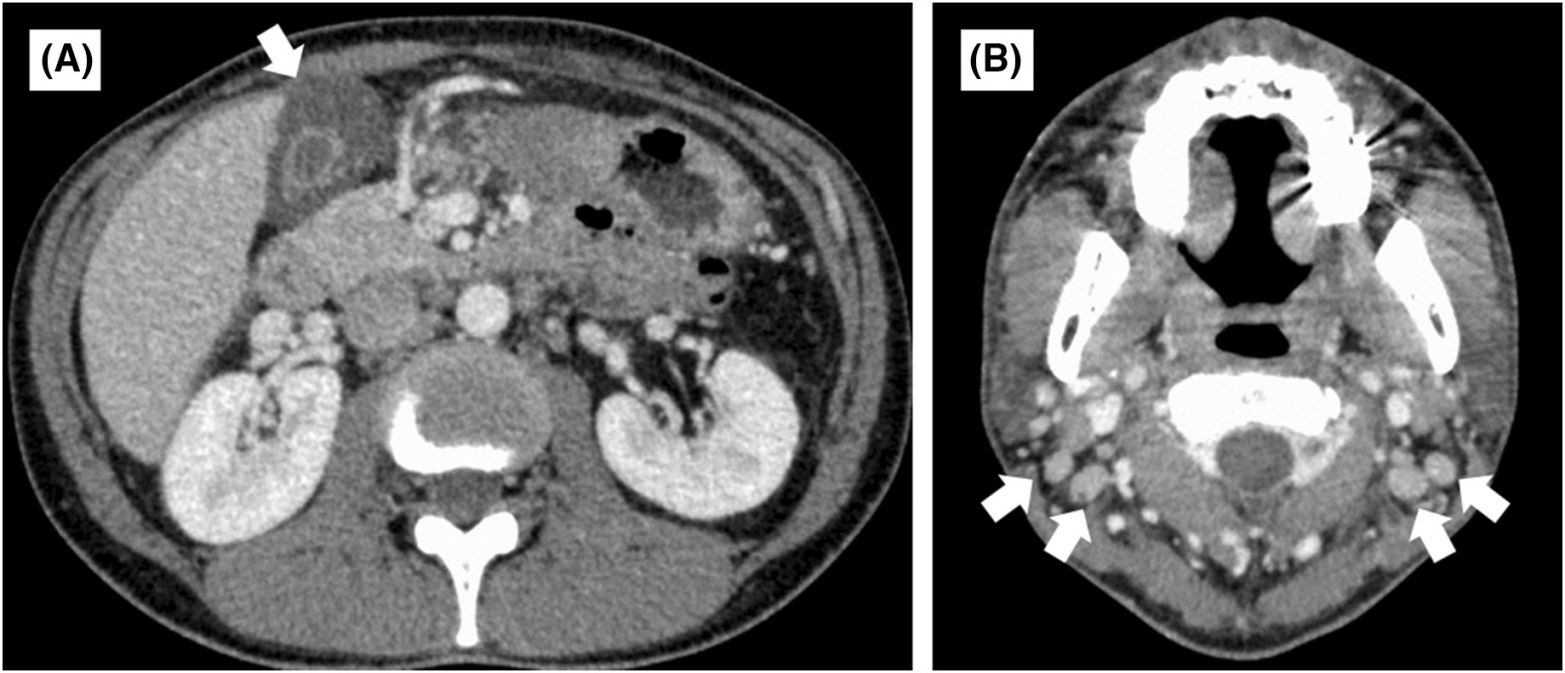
Findings of chest and abdominal computed tomography with contrast enhancement. Chest and abdominal computed tomography with contrast enhancement shows a markedly thickened gallbladder wall measuring 12 mm (A, arrow) and multiple enlarged cervical lymph nodes (B, arrows).
